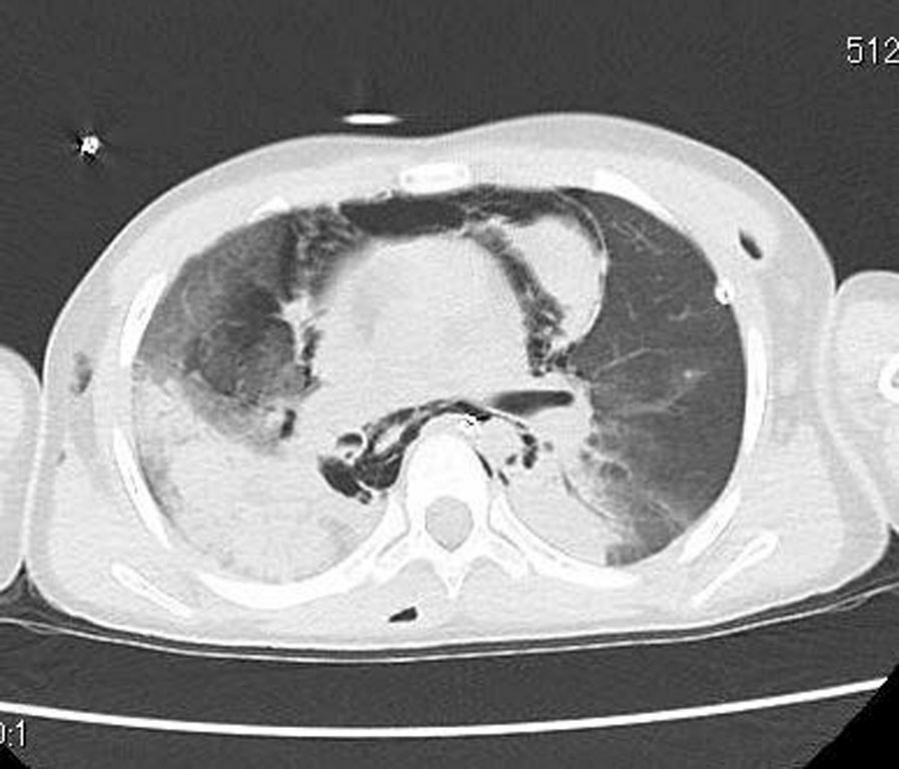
Computed tomography image obtained before surgery showing injury of the tracheal bifurcation, and the right and left main bronchi
